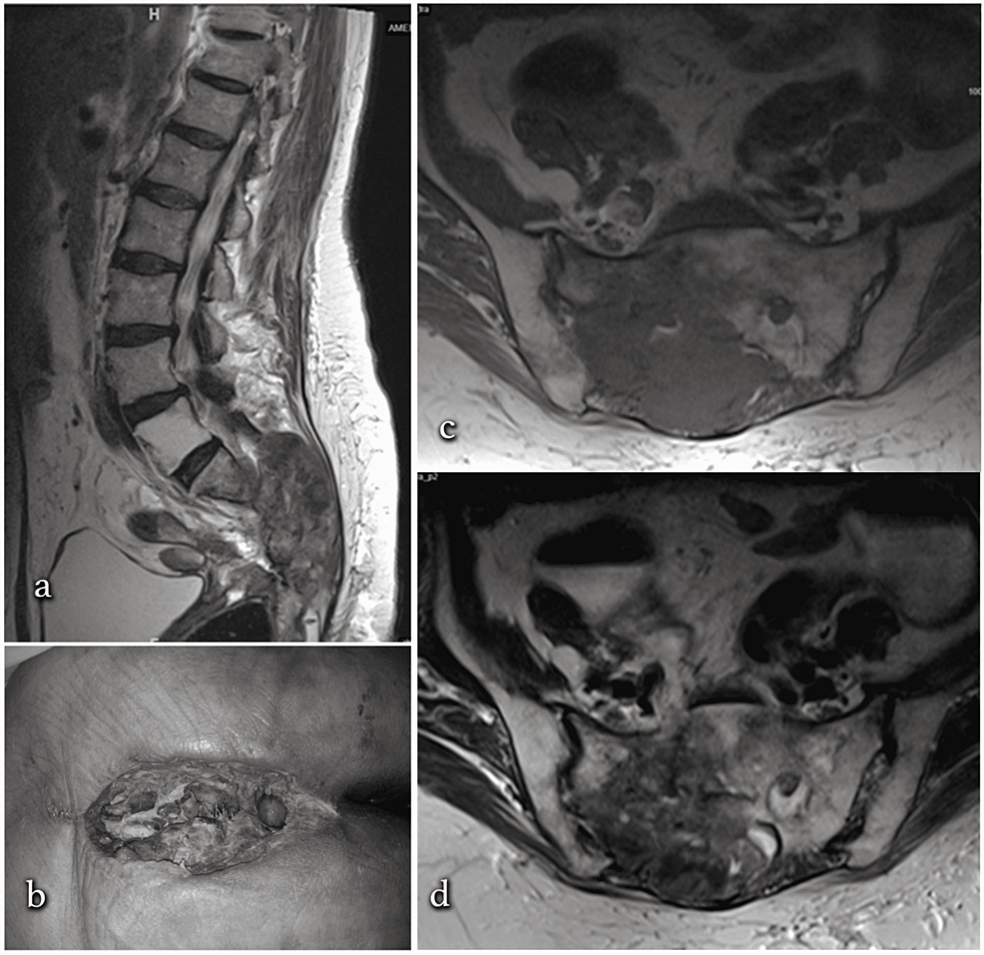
Abscess developing after sacral chordoma excision a) T2-weighted sagittal MRI image. b) Eventration of the skin surface in the infected area. c) T1-weighted axial MRI image. d) T2-weighted axial MRI image MRI: magnetic resonance imaging
